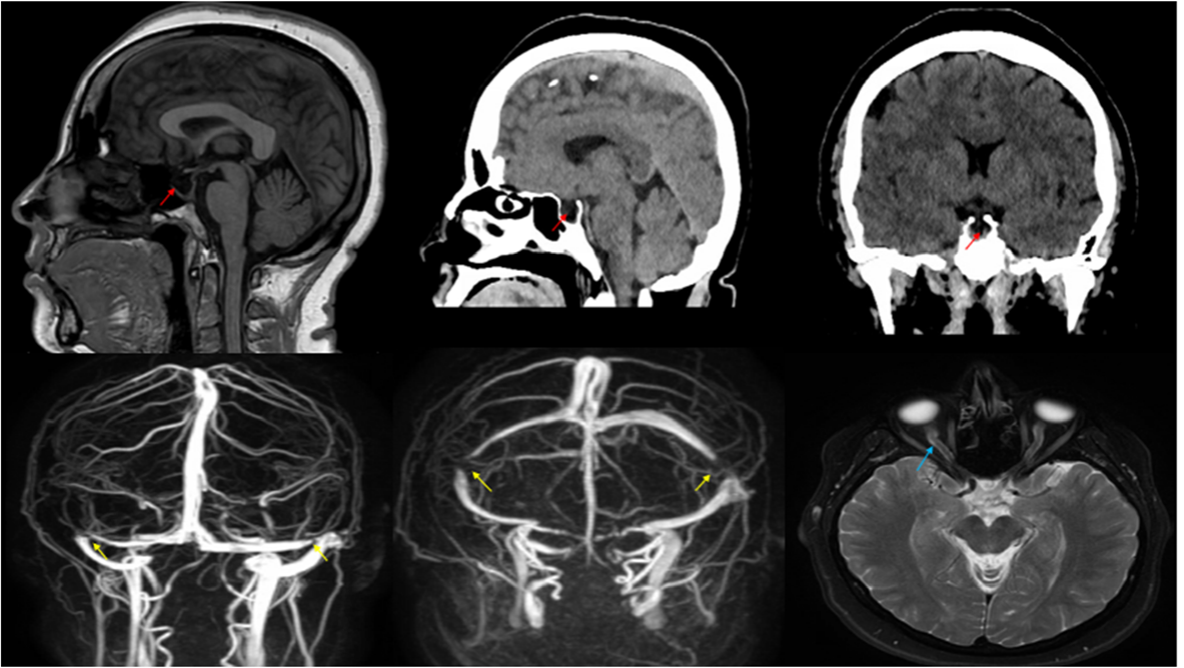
Top*: A computed tomography scan of the head in the top middle picture showed pontomedullary hypodensity, and a partially empty sella turcica, noting the top first one on the left is brain MRI (red arrows). *Bottom*: Brain magnetic resonance imaging with magnetic resonance venography revealed a stenosis in the lateral aspect of the transverse sinus (*yellow arrows*), and perioptic nerve sheath distention which is consistent with papilledema (*blue arrow*)
